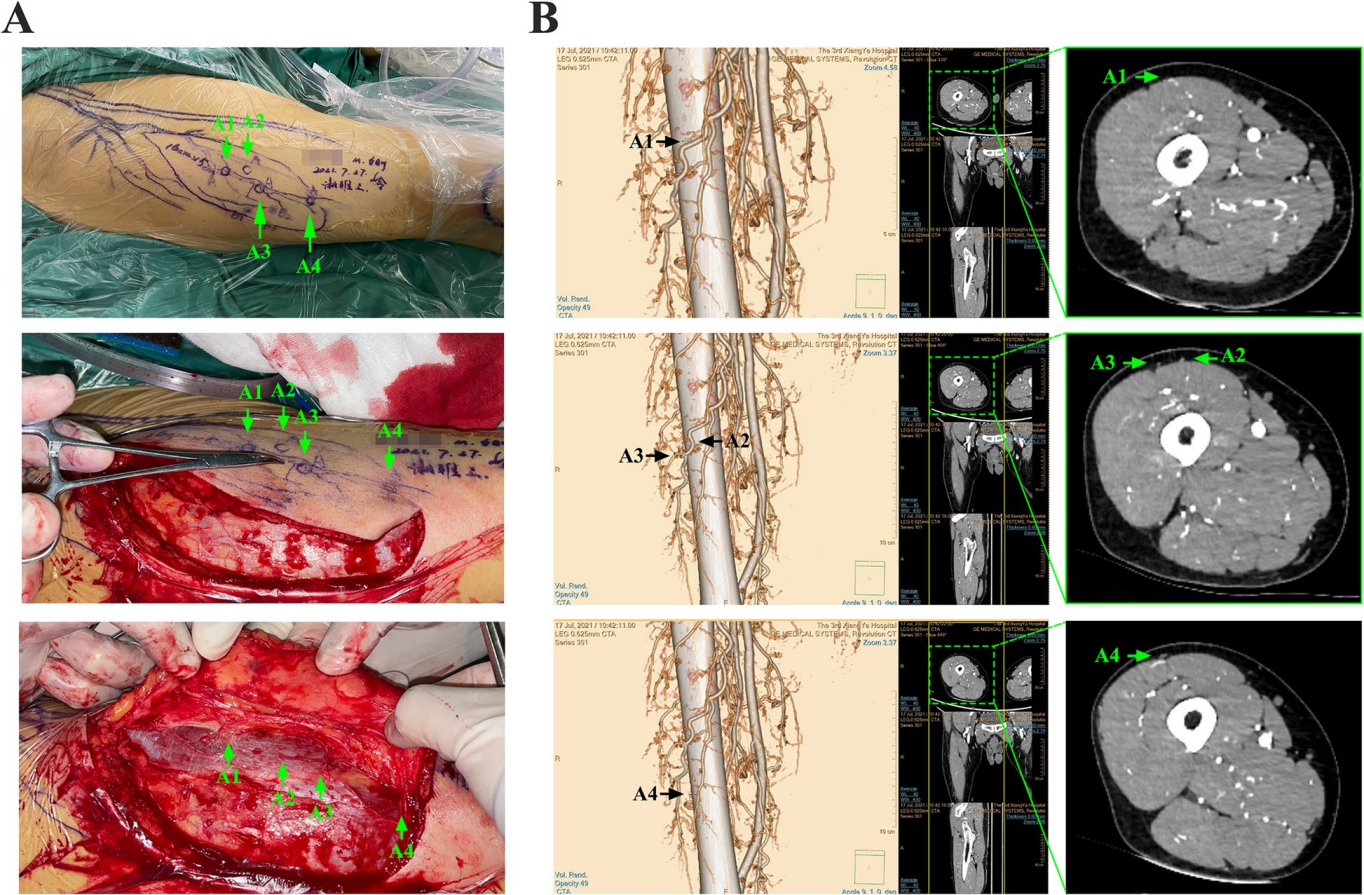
The ALTP flap was obtained intraoperatively by mapping the emerging points. A The four emerging points A1-A4 were depicted pre-operation, and verified intra-operation. B These emerging points were firstly found in transverse slice (on the rightmost), and then they were marked in three plane and 3D CTA images automatically. Identity information has been removed to ensure patient anonymity
